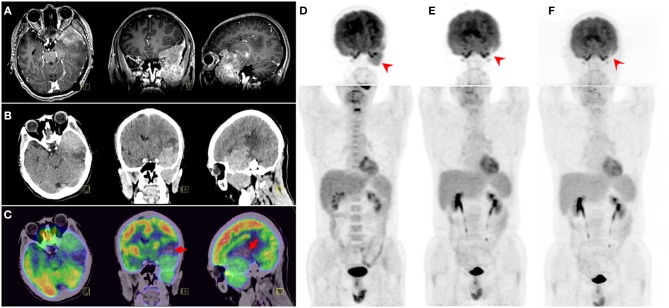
MRI revealed a heterogeneous enhanced mass lesion with meningeal and adjacent frontal-temporal lobes involvement (A). CT displayed a heterogeneous hyper-intensity mass lesion and peripheral edema (B). 18F-fluorodeoxyglucose (^18^F-FDG) PET/CT showed intense FDG uptake with SUV_max_ of 12.0 in the left frontal–temporal lobes. Part of the lesion was naive with FDG uptake (arrows) (C), which could be caused by possible intratumoral hemorrhage. Adjacent skull base bone destruction and extracranial extension to the left infratemporal fossa were also observed. Maximum intensity projection (MIP) view of pretreatment ^18^F-FDG PET/CT (D) showed the solitary bulky mass. Two consecutive ^18^F-FDG PET/CT follow-ups (E,F) demonstrated complete remission.
